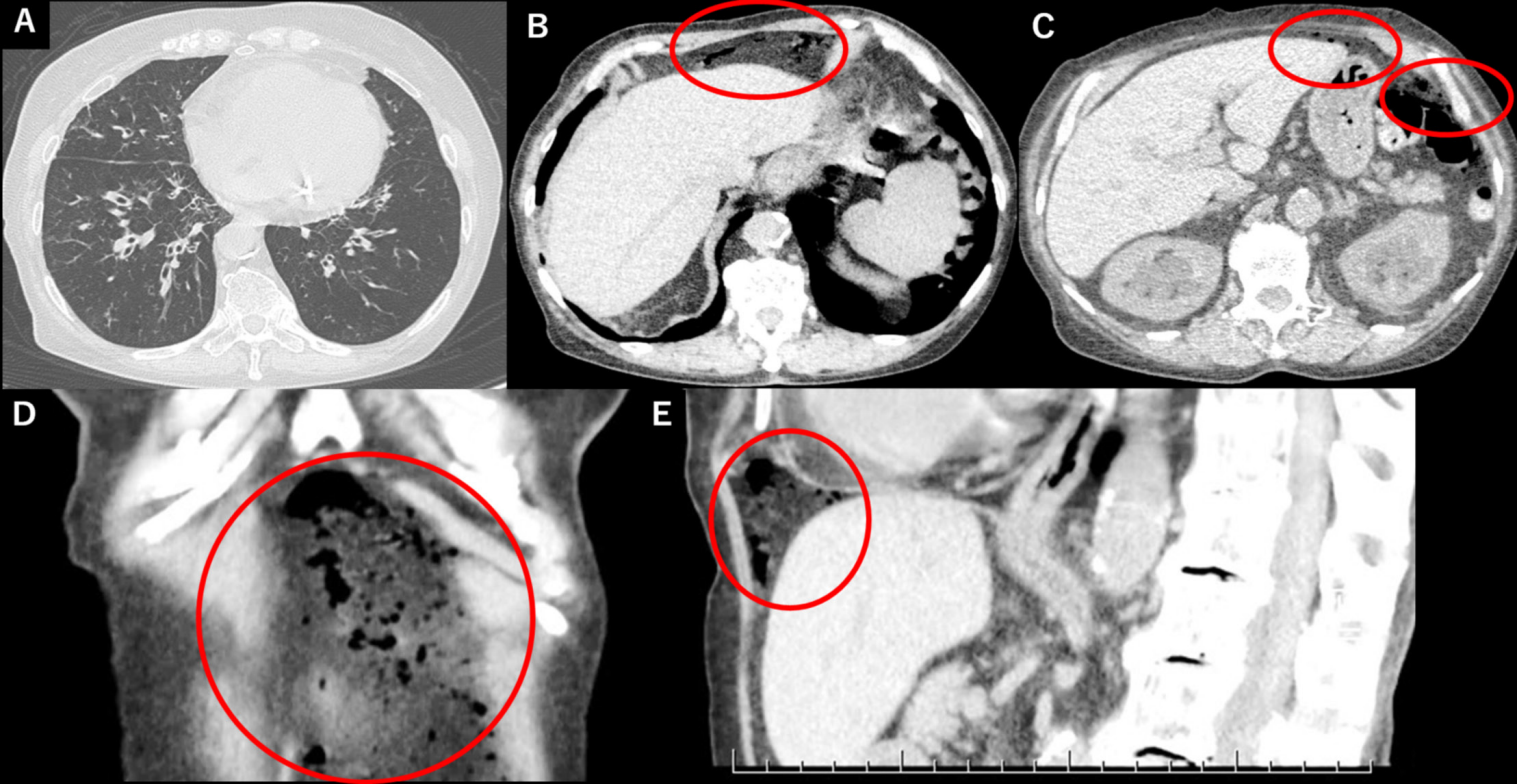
Initial computed tomography (CT) images on admission. (A) Axial chest CT in lung window showing diffuse bronchial‐wall thickening, consistent with asthma exacerbation. (B, C) Axial, (D) coronal, and (E) sagittal abdominal‐window CT images show localised intraperitoneal free air near the diaphragm (circles). No evidence of pneumothorax, pneumomediastinum, or intra‐abdominal pathology is observed.
